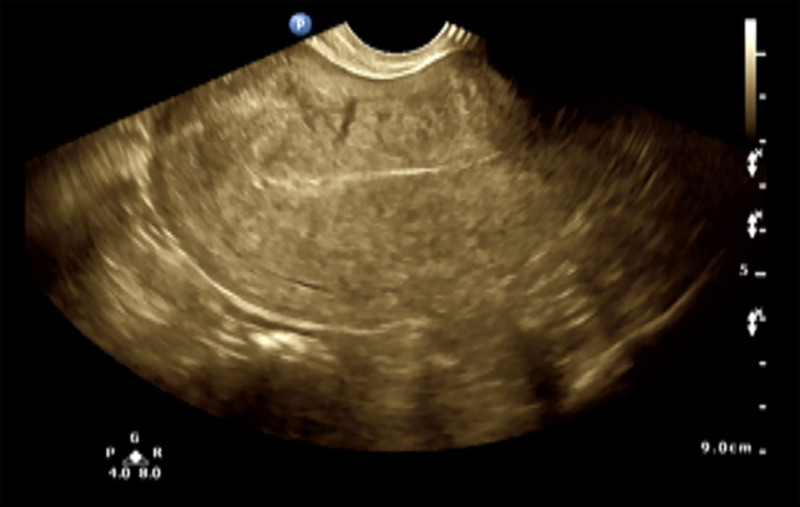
Gynecological ultrasound image of Patient 1.
